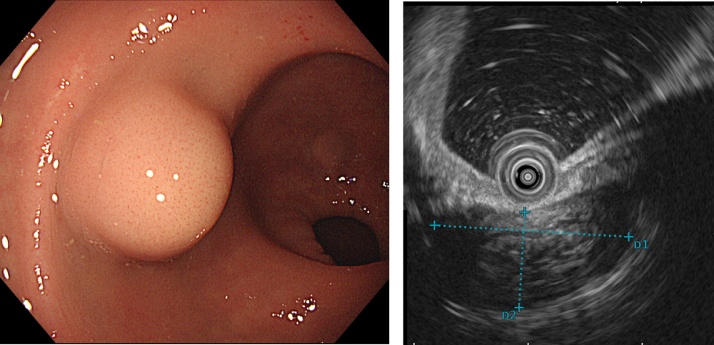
Colonoscopy finding. Colonoscopy showed 20-mm of sub-mucosal tumor at low rectum (Fig. 1a). The tumor was elastic hard and move easily. An endoscopic ultrasonography revealed low echoic mass (Fig. 1b). Biopsy revealed no specific findings.
